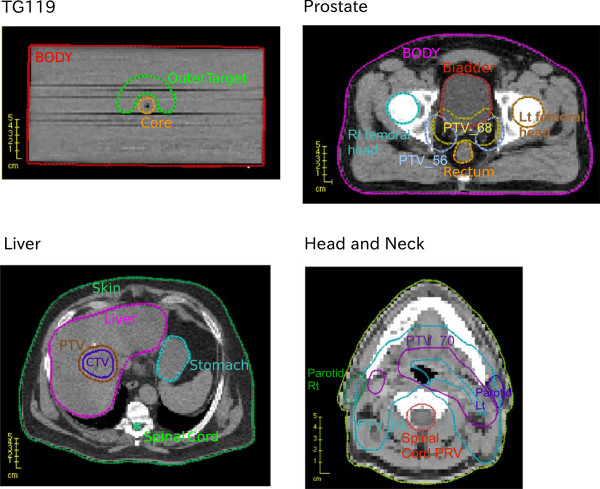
Axial views of the four cases. CT and structures for the four cases for a representative CT slice (which shows some but not all of the structures included in the dataset).
